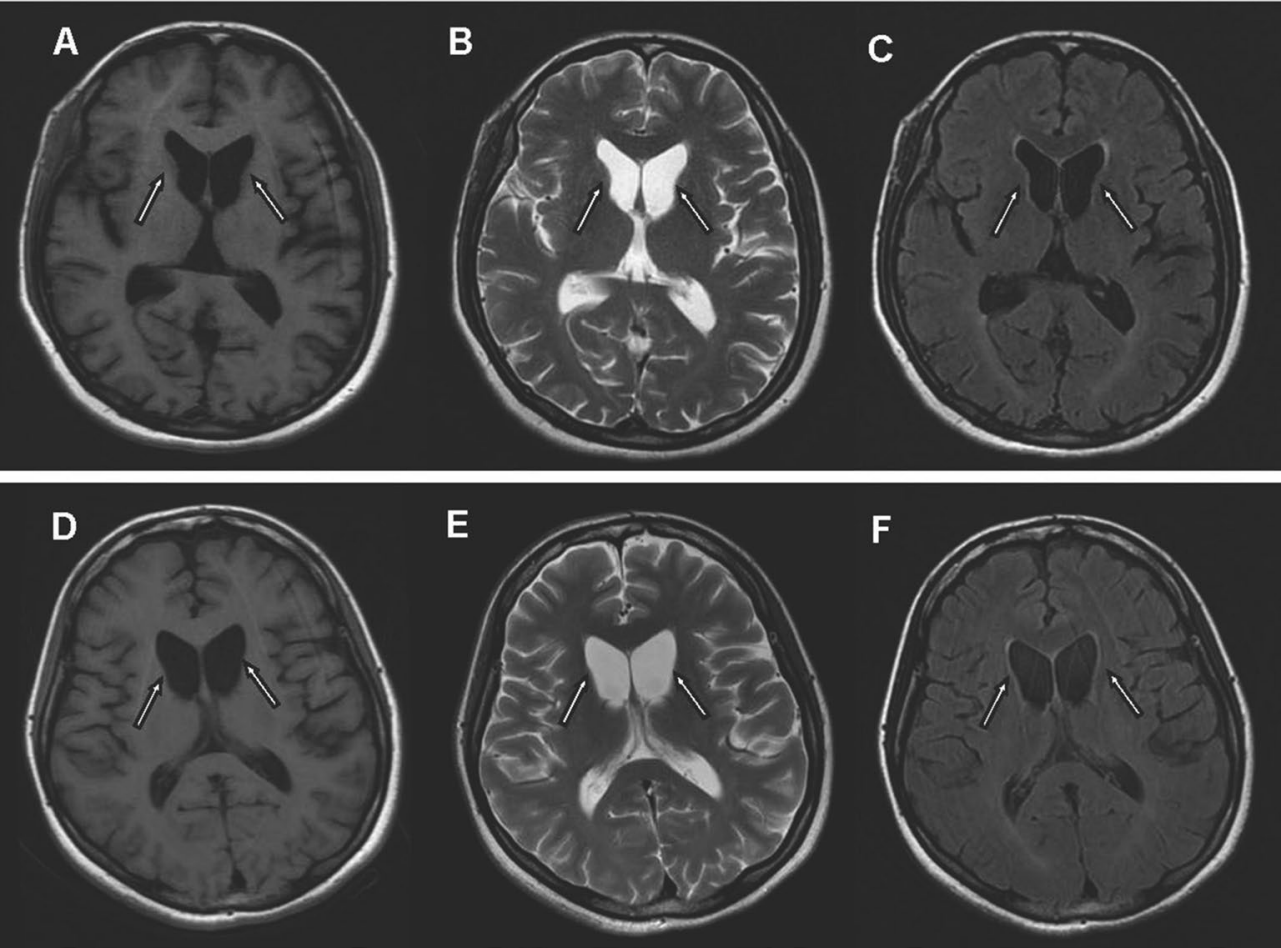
Magnetic resonance images show bilateral atrophy of the head of the caudate nucleus and anterior horn dilation of lateral ventricles (arrows). (A and D: T1-weighted image, B and E: T2-weighted image, C and F: Fluid-attenuated inversion recovery image, A, B and C of patient 1, D, E and F of patient 2)
